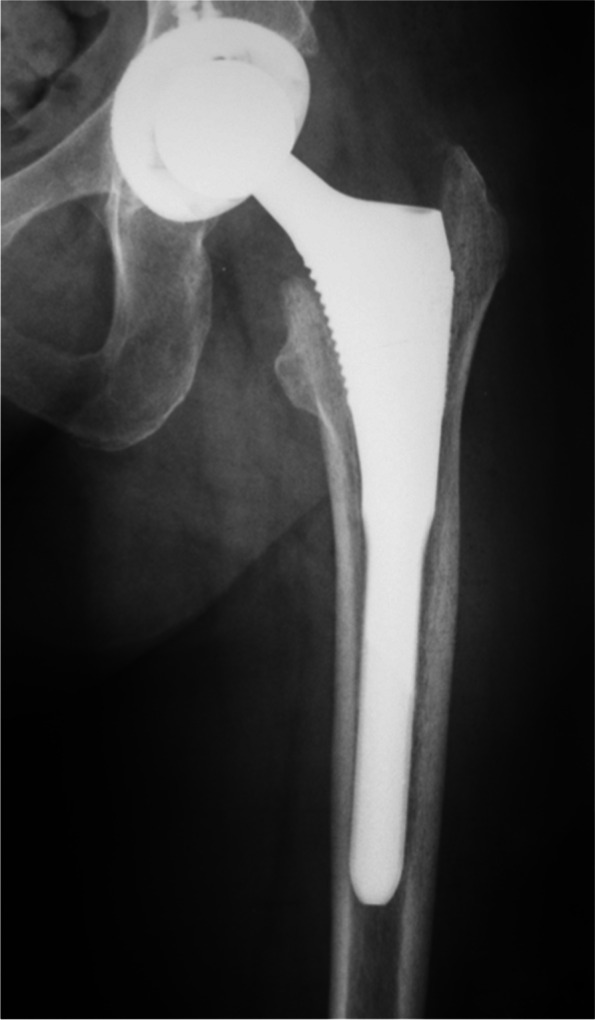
This is a representative postoperative radiograph demonstrating a well-fixed modular femoral stem
